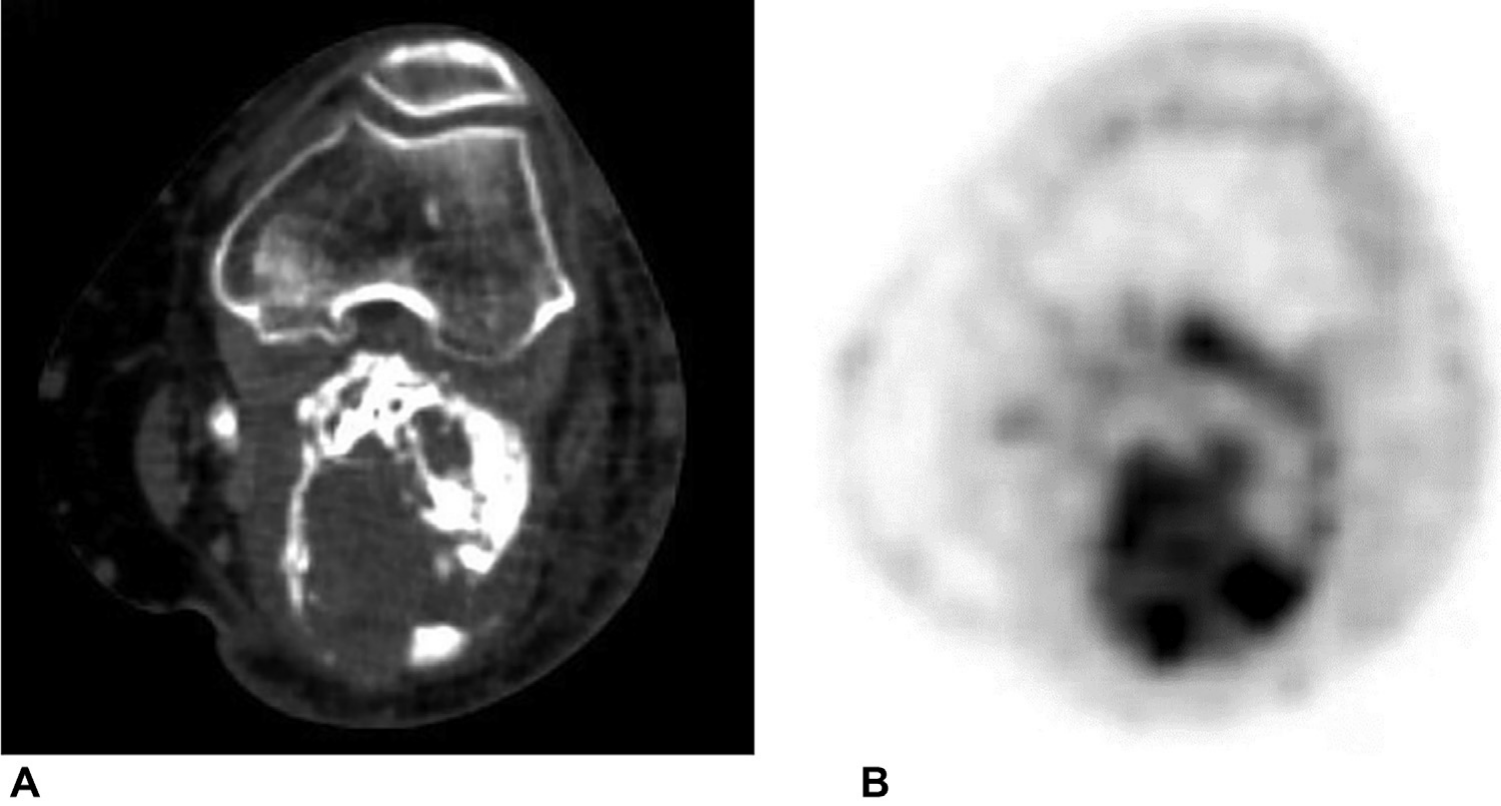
FDG PET/CT scan showing inhomogenous uptake of radioisotope predominantly in the soft-tissue areas of the mass.
